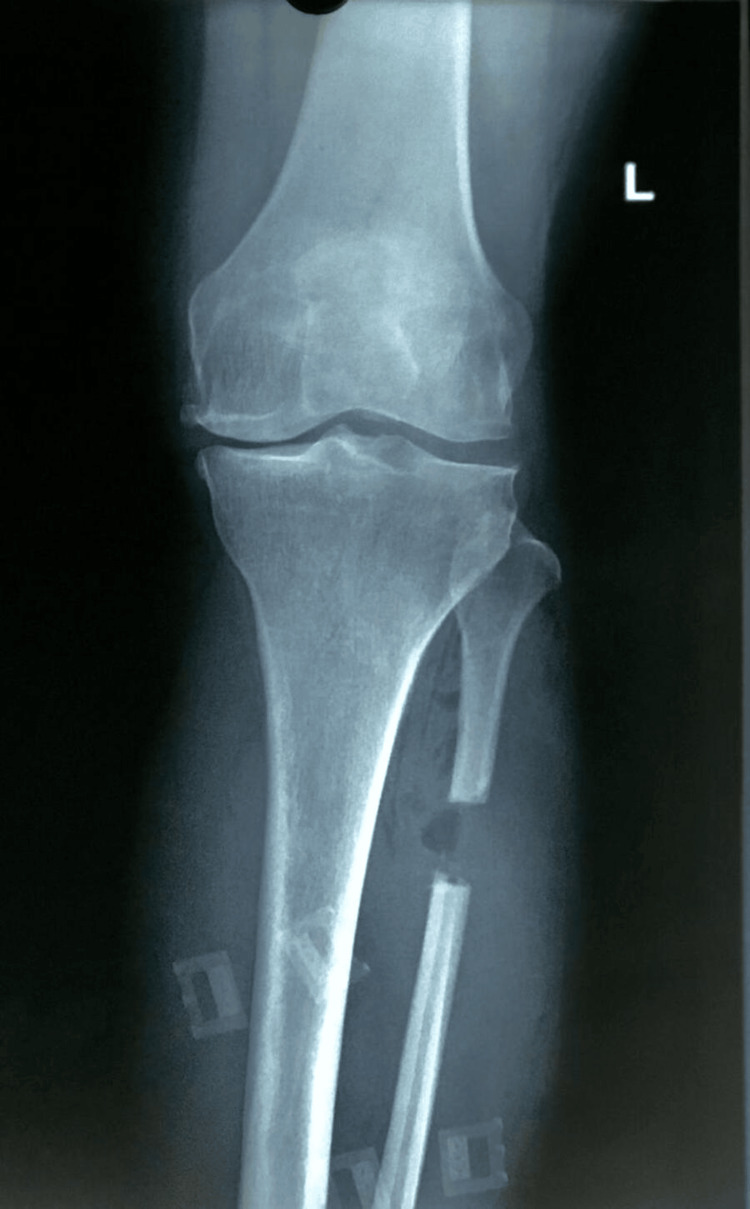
Postoperative radiograph demonstrating medial joint space widening
